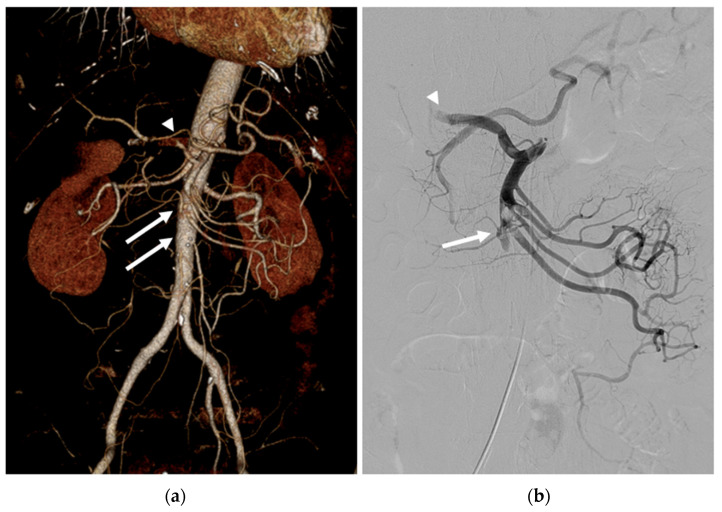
(a) Three-dimensional volume-rendered image of abdominal CT angiography revealed a segmental thrombus in the proximal SMA (arrows) and a focal thrombus in the CHA (arrowhead). A hepaticomesenteric common trunk was also observed. (b) Initial angiography revealed occlusion of the proximal SMA (arrow) and the CHA (arrowhead).
